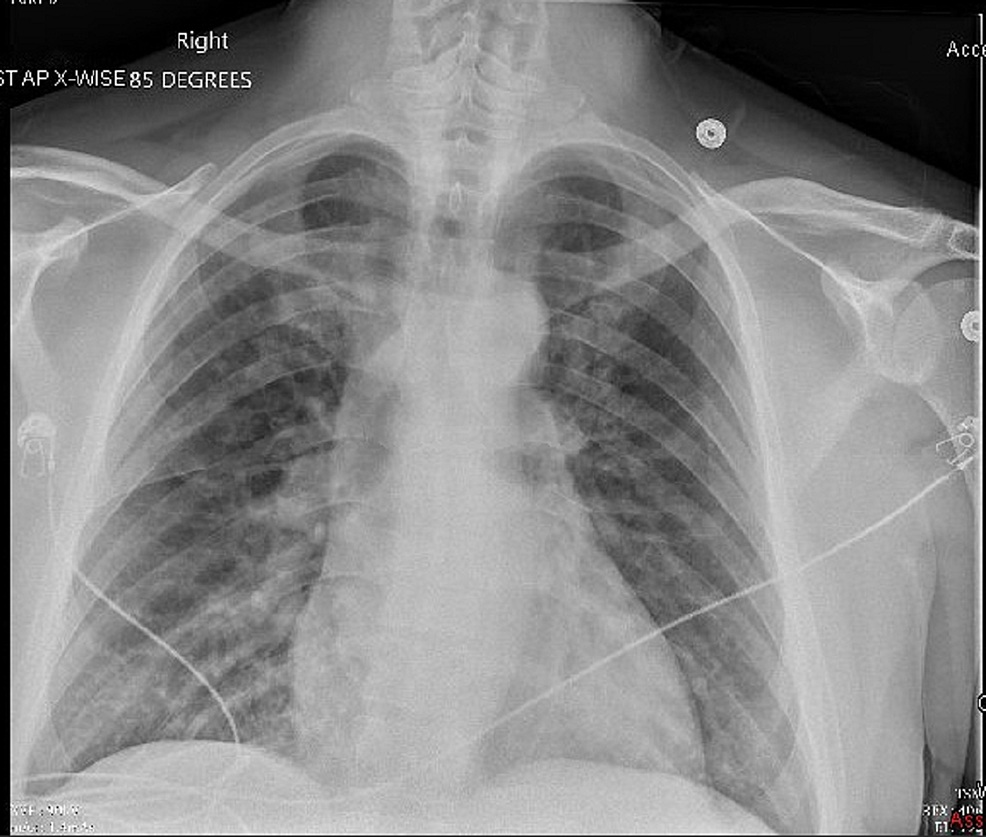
Chest x-ray (CXR) showed diffuse patchy lung infiltrates, concerning possible venous congestion or pulmonary edema with enlargement of the cardio-mediastinal silhouette and double density knob sign
